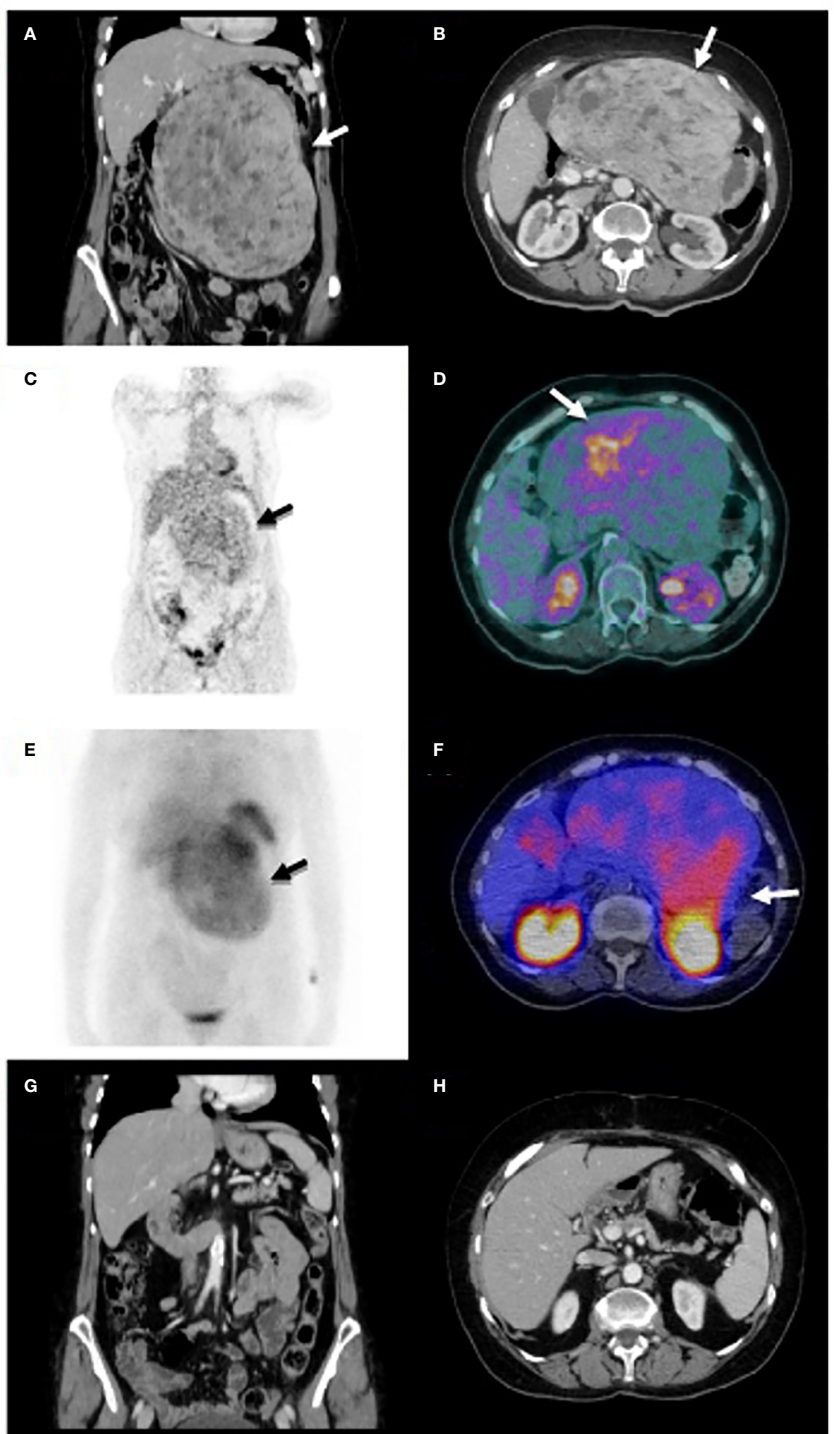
Coronal and axial CT views at diagnosis (A, B) show an abdominal solid mass (23x13x18 cm), with several necrotic-cystic zones, closely located to the lesser curvature of the stomach, which displaced the duodenum, pancreas, aorta and cave vein. Coronal and axial images of 18-FDG PET/CT scan (C, D) reveal increased metabolic activity in the whole mass. Coronal and axial images of somatostatin receptor scintigraphy SPECT/CT show increased expression of somatostatin receptors (E, F). Coronal and axial images of CT after surgery show complete tumor resection (G, H).
